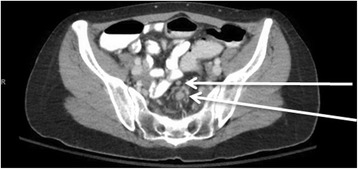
Preoperative CT scan demonstrating multiple, borderline, perirectal lymph nodes with the largest measuring 1.0 cm
